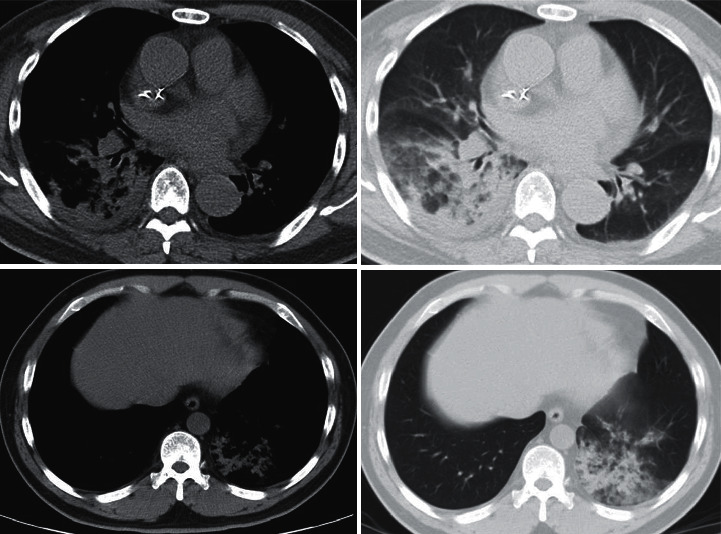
CT images of 2 neonates with NBP.
